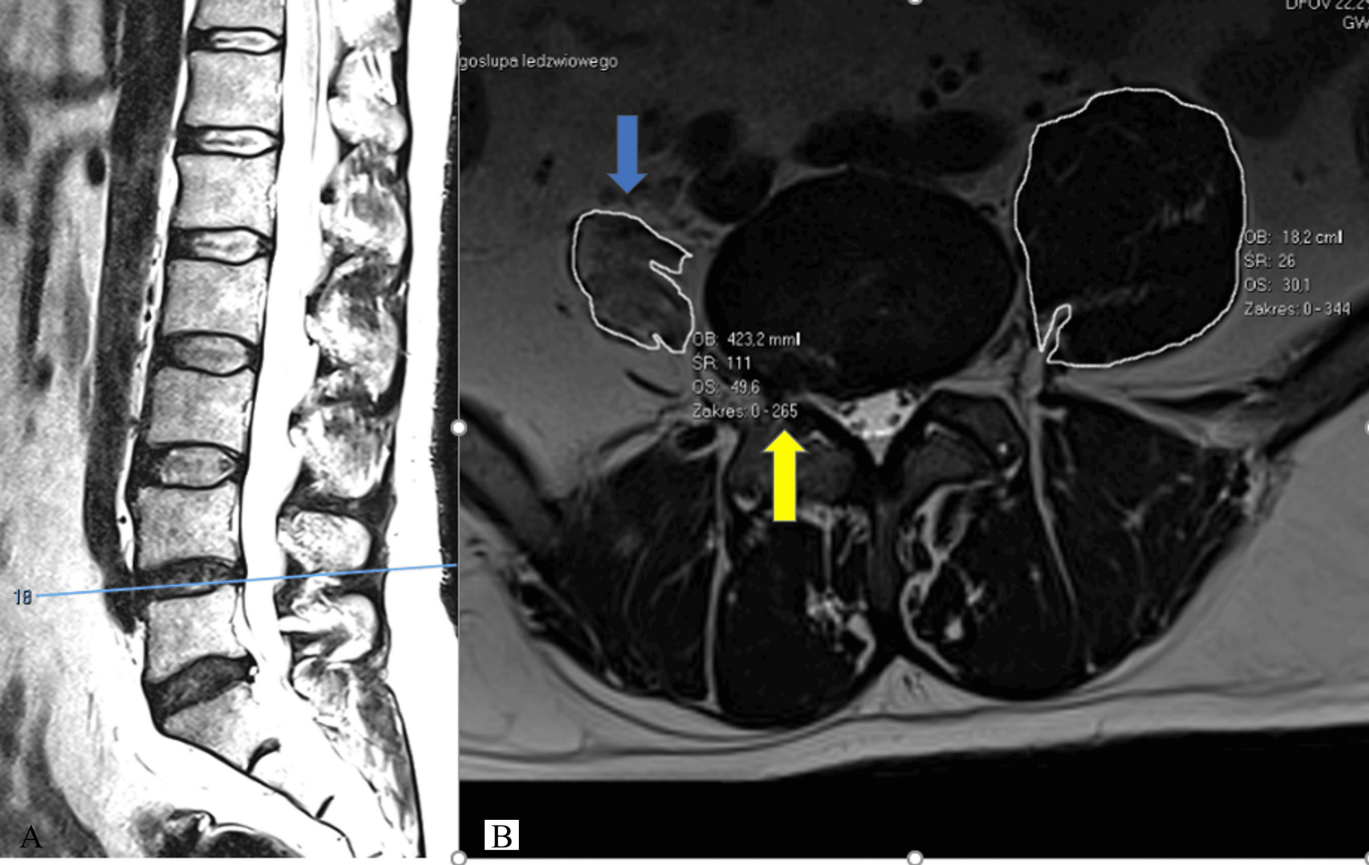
Sagittal and Axial T2-Weighted MRI at the L4/L5 Level A: Sagittal T2-weighted MRI showing the midline levels of L4/L5 and L5/S1, with Pfirrmann grade II degeneration (“black disc”) at both segments.
